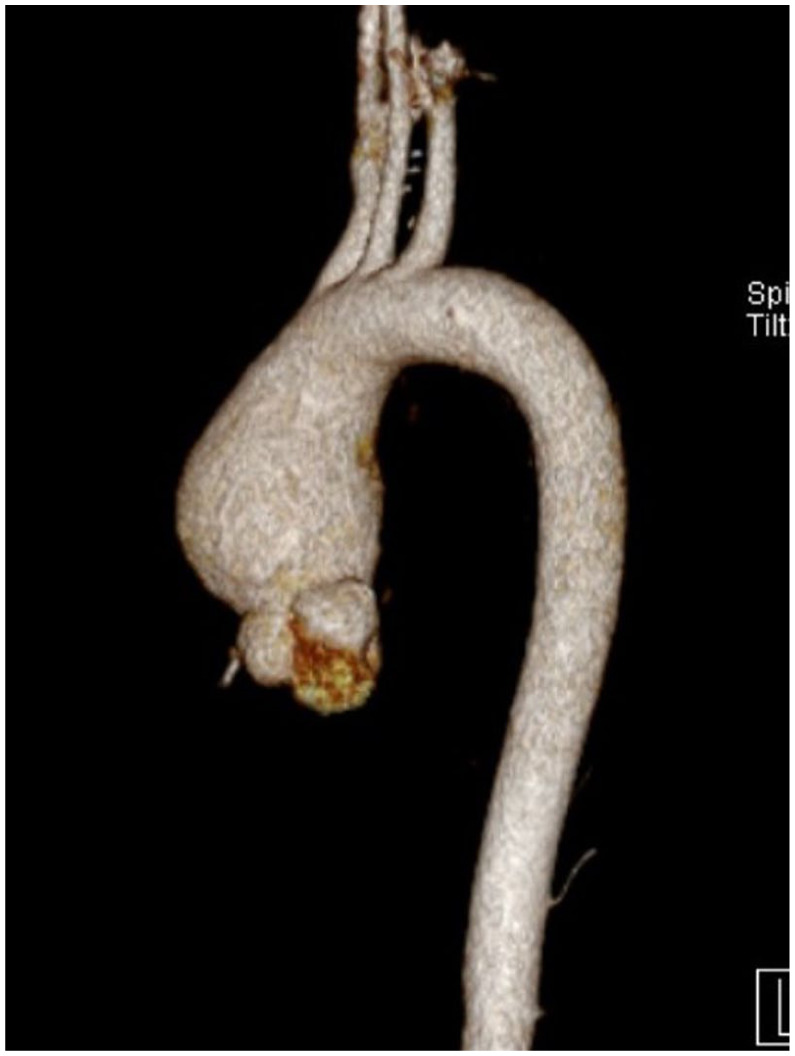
3D view of aortic aneurysm on initial CT scan.
